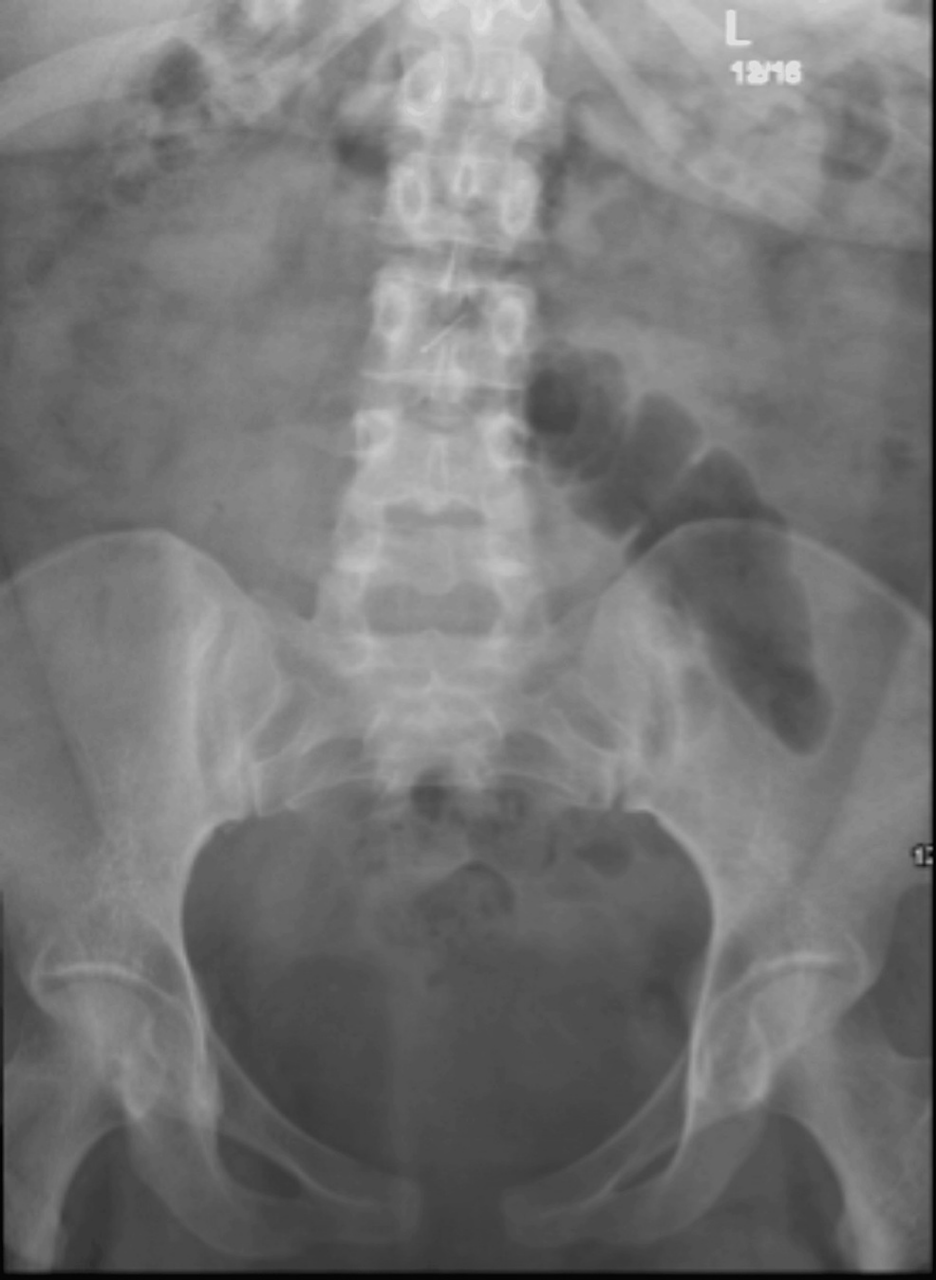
X-ray of the spine. The missing epidural catheter was not detected by X-ray.
